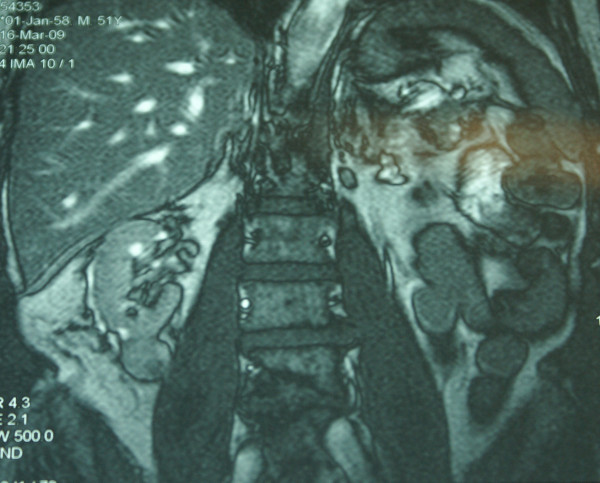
Magnetic resonance imaging T2 (MRI) scan also showing a 2,5 × 2,7 cm mass at the lower pole of the right kidney and an adenoma of the right adrenal gland.
